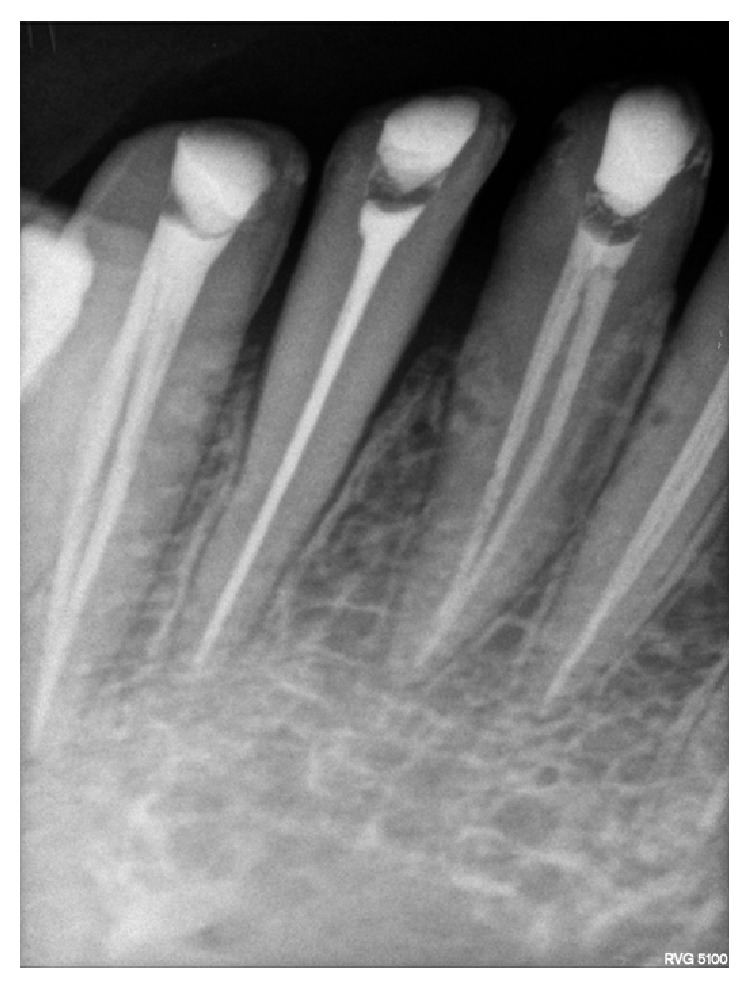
Radiograph showing obturation in two separate canals in 32 and 41.
